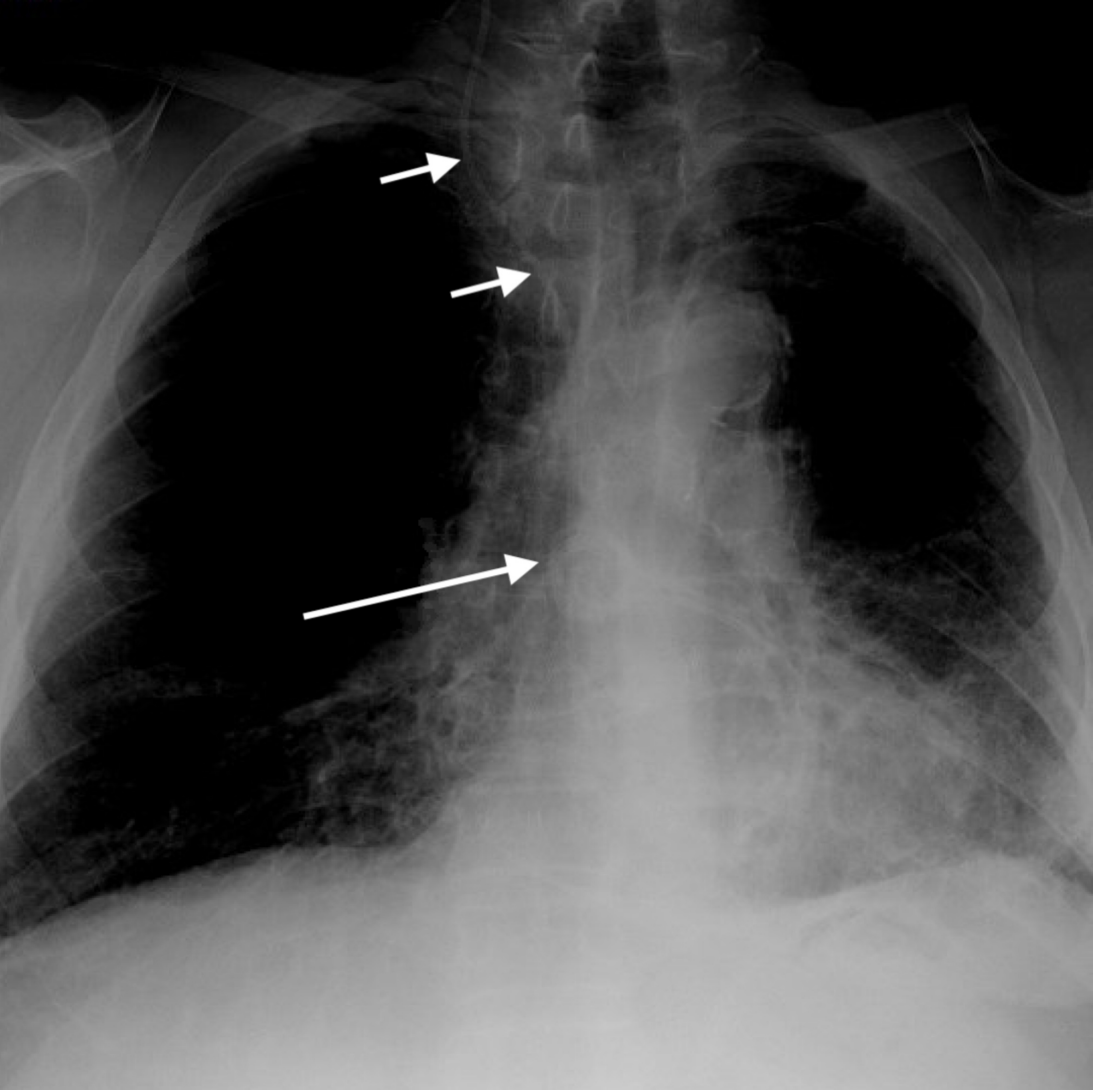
Anteroposterior chest X-ray performed as part of a shunt series demonstrates migration of the distal catheter of the ventriculoperitoneal shunt through the superior vena cava (short arrows) and coiling into the right heart (long arrow).
